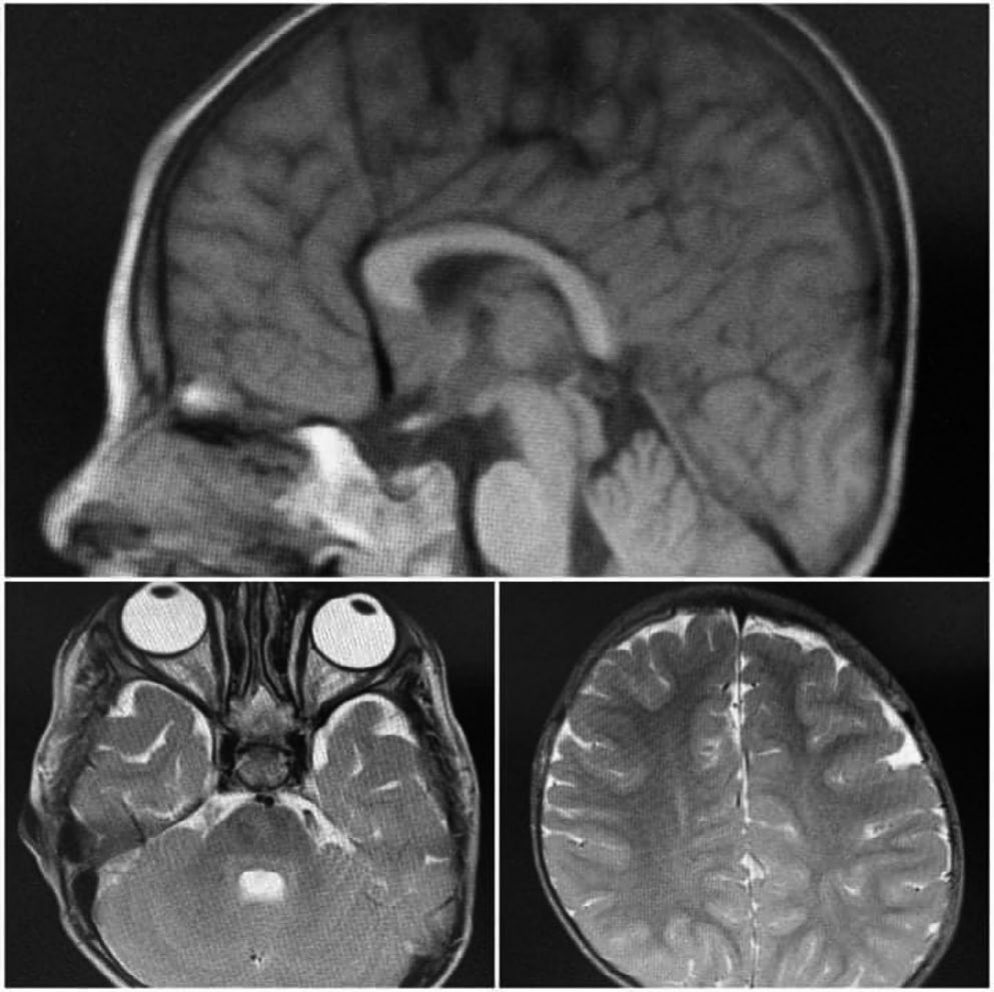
Brain imaging (MRI) shows evidence of mild cerebral atrophy and mild thinning of the corpus callosum with mild bilateral retinal coloboma
